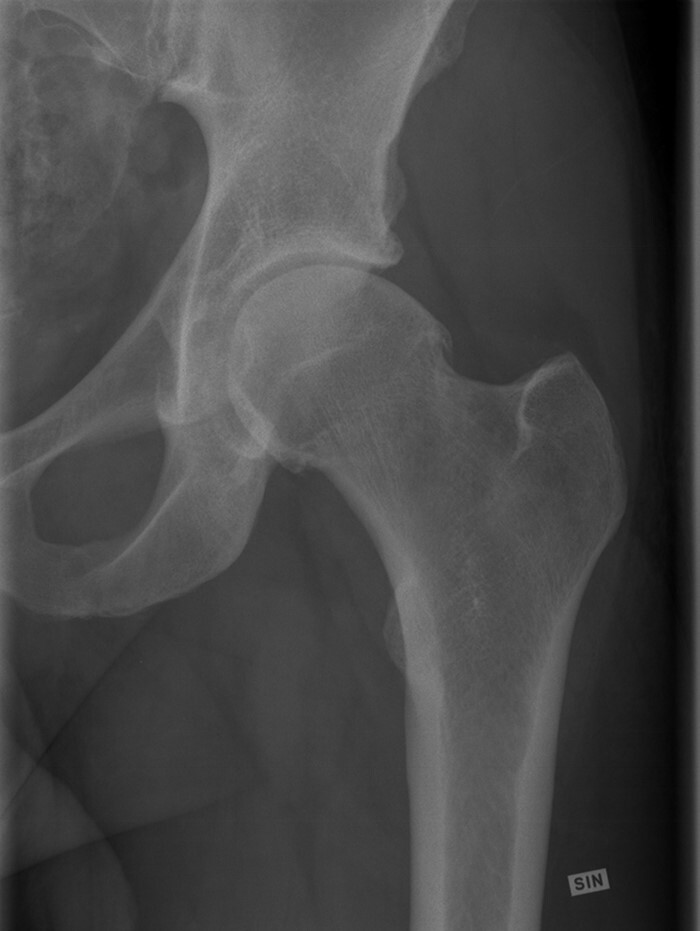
Close-up views taken from the anteroposterior pelvic radiographs showing a 35-year-old ice hockey player with cam morphology (α-angle 74°) and early osteoarthritis leading to total hip arthroplasty 15 years later.
